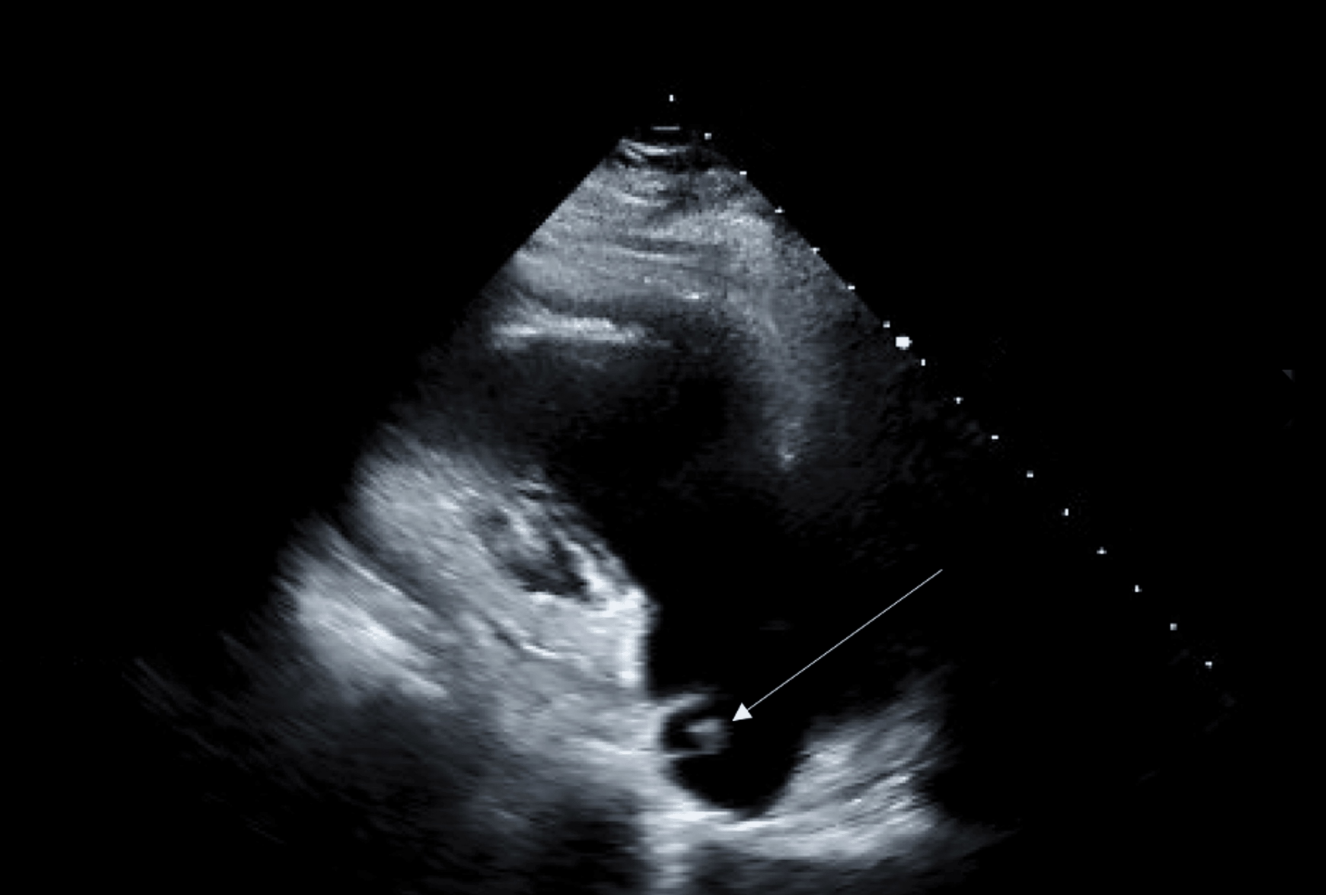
TTE showing a lesion in the right atrium (marked by arrow) TTE: transthoracic echocardiogram
